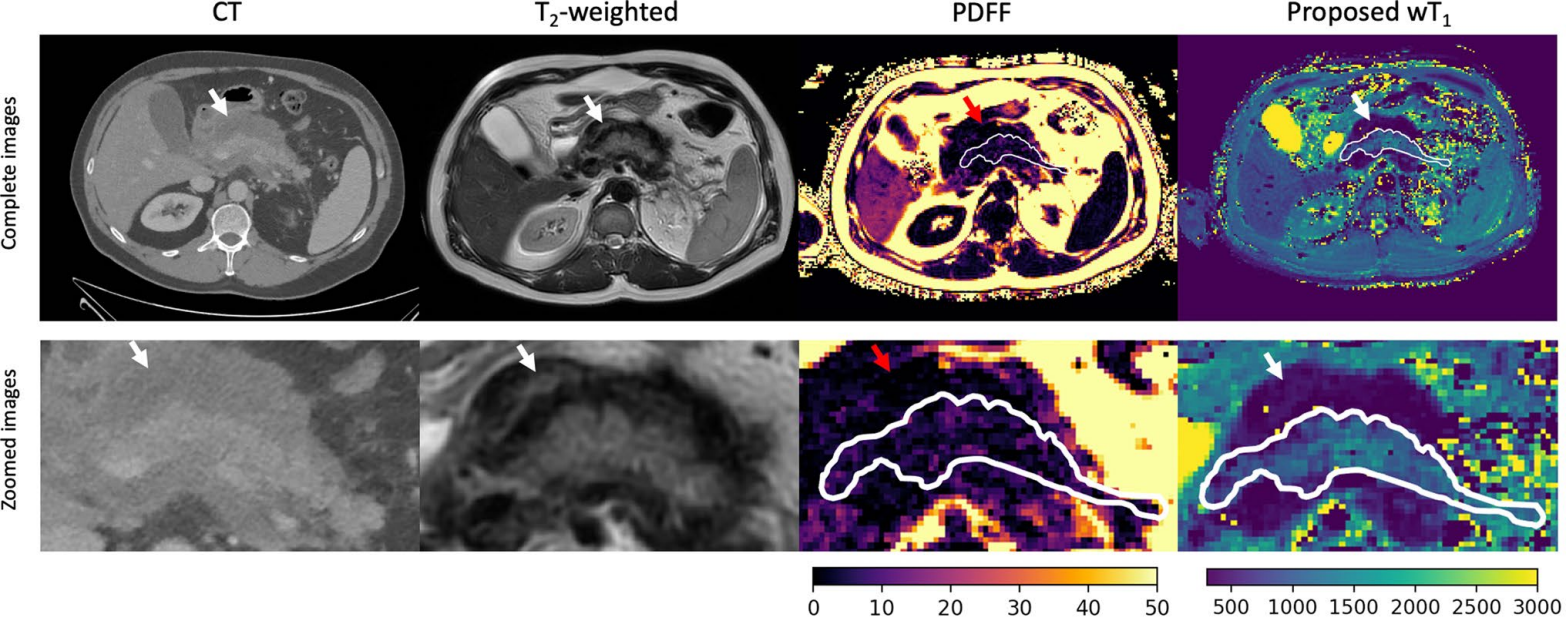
Comparison between clinical images and wT_1_ maps for a necrotizing pancreatitis case. A 46-year-old woman presented alcohol-induced necrotizing pancreatitis. As part of the clinical routine imaging of the patient, an axial contrast-enhanced CT scan during the portal phase was acquired. T_2_-weighted images, PDFF and wT_1_ maps were acquired on the same day as the CT scan. The displayed CT image, T_2_-weighted image and PDFF map demonstrate heterogeneous enhancement of the pancreatic parenchyma with poorly defined contours, surrounded by a heterogeneous fluid collection suggestive of walled-off necrosis (arrow). Moreover, the pancreas parenchyma (white contour) exhibits fat content as displayed by the PDFF map. This underscores the importance of the water-fat separation in accurately estimating T_1_ values in the pancreas. Remarkably, the wT_1_ map provides a detailed depiction of the pancreatic anatomy, differentiating necrotic tissue from healthy parenchyma with greater contrast than both the CT image and the PDFF map
